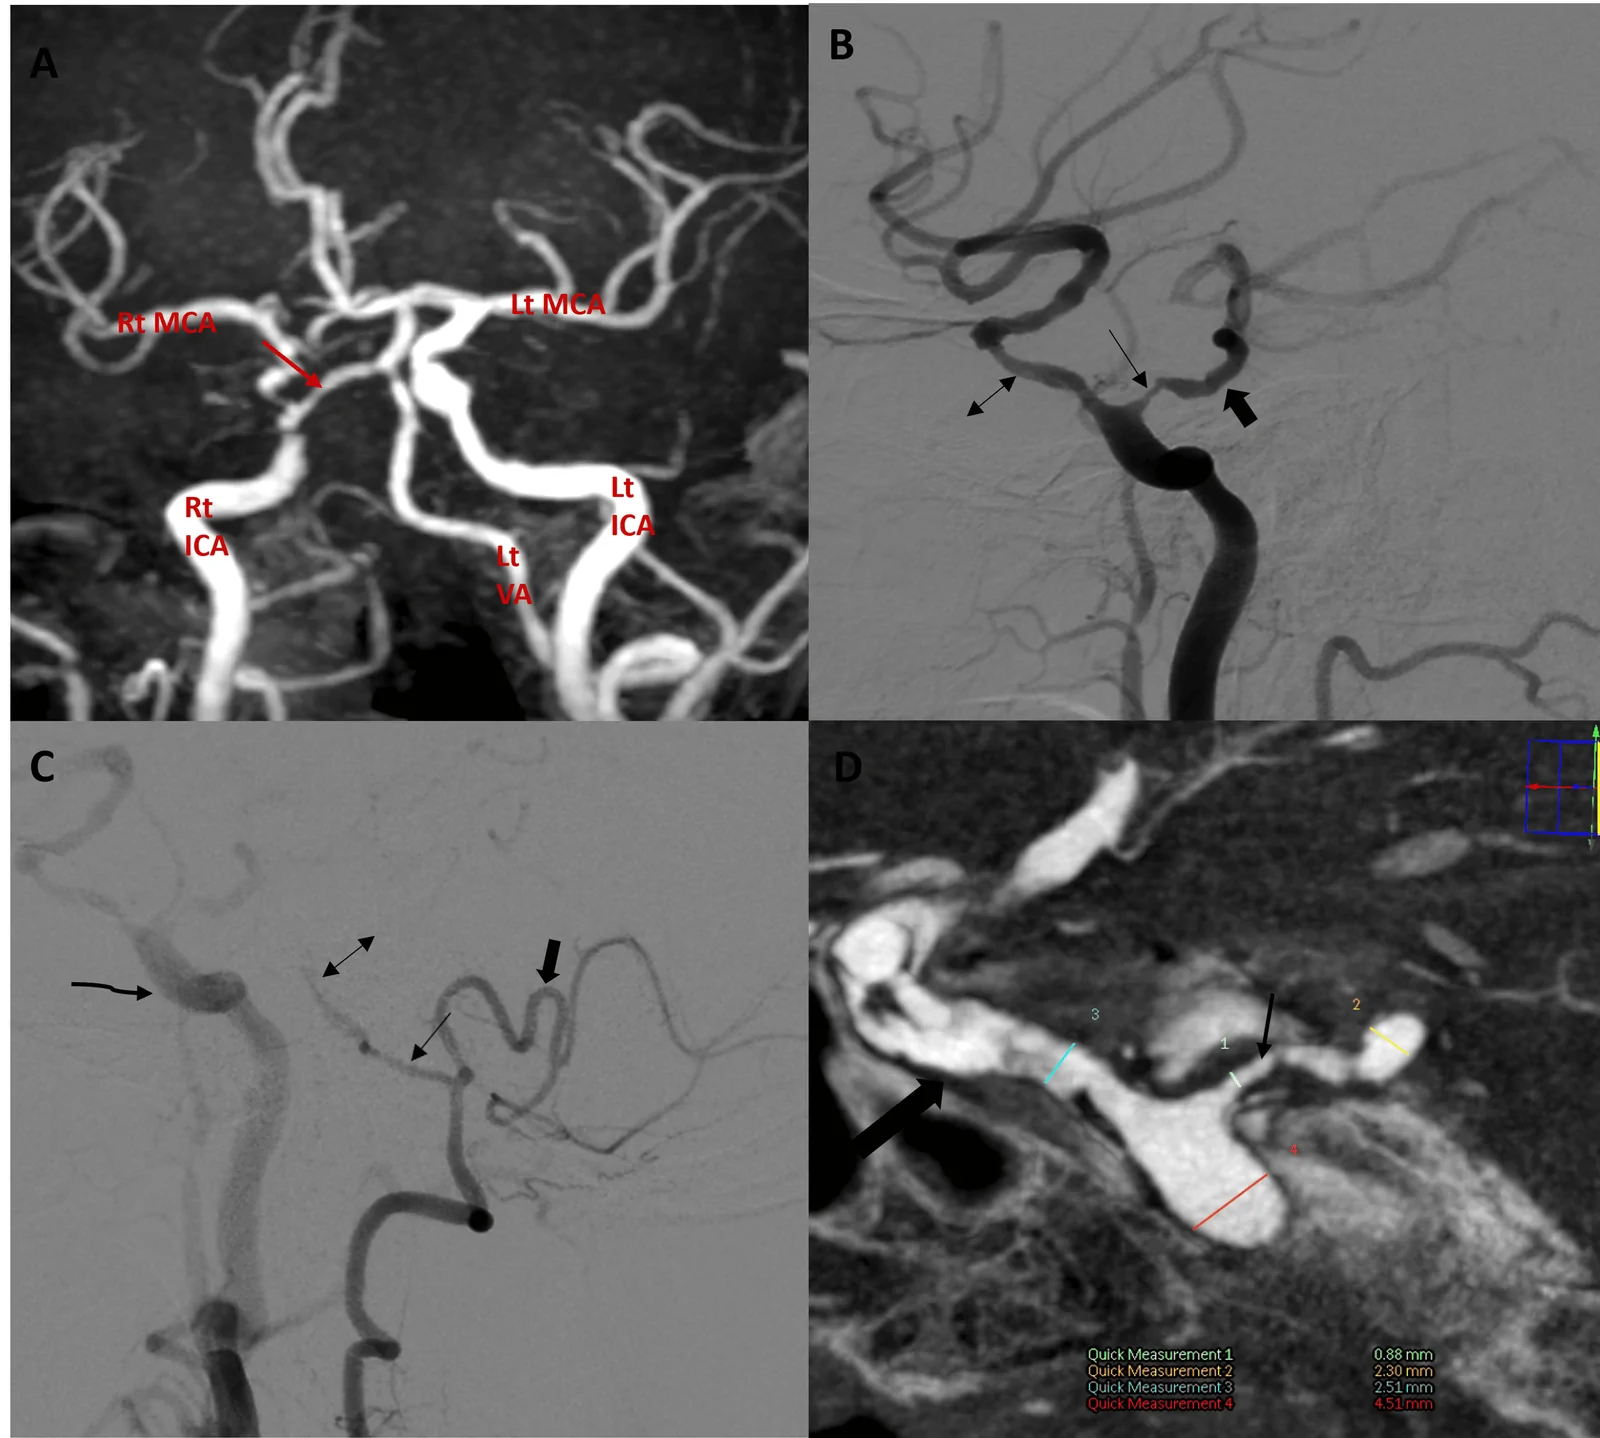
Preprocedural vascular imaging (A) Time-of-flight magnetic resonance angiography demonstrates a Saltzman type I persistent primitive trigeminal artery (arrow) with ostial stenosis and coexisting right cavernous internal carotid artery stenosis. (B) Lateral right internal carotid angiography demonstrates severe short-segment ostial stenosis (long arrow) of the persistent primitive trigeminal artery (block arrow) and long-segment stenosis of the cavernous internal carotid artery (double-headed arrow). (C) Lateral right vertebral angiography demonstrates a diminutive vertebral artery (arrow) with minimal contribution to the basilar artery (double-headed arrow). The curved arrow indicates the right internal carotid artery, and the block arrow indicates the posterior inferior cerebellar artery. (D) Cone-beam CT angiography demonstrates irregular luminal narrowing of the right cavernous internal carotid artery (block arrow) and severe ostial-proximal stenosis of the persistent primitive trigeminal artery (arrow). Measurements confirm severe stenosis according to the Warfarin-Aspirin Symptomatic Intracranial Disease (WASID) method [5]. ICA: Internal Carotid Artery, MCA: Middle Cerebral Artery, Rt: Right, Lt: Left, VA: Vertebral Artery.
